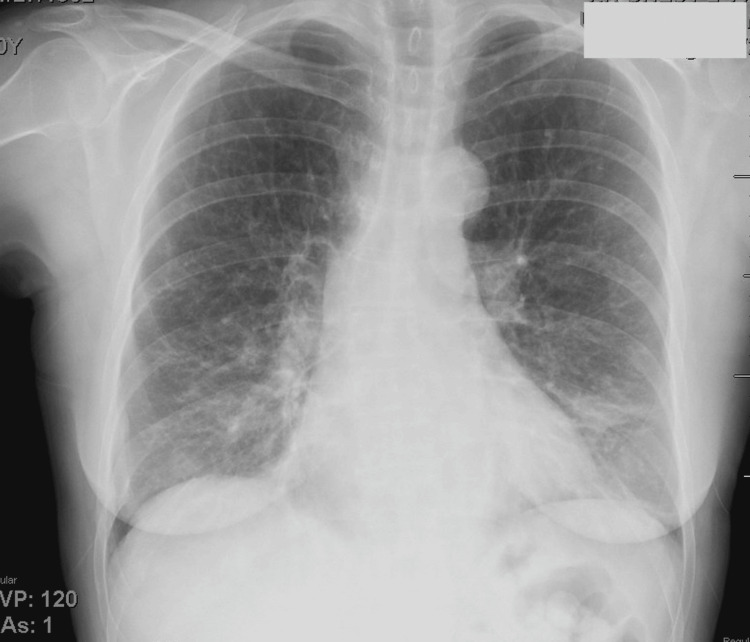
Chest radiograph demonstrating bilateral lower lobe opacities.
